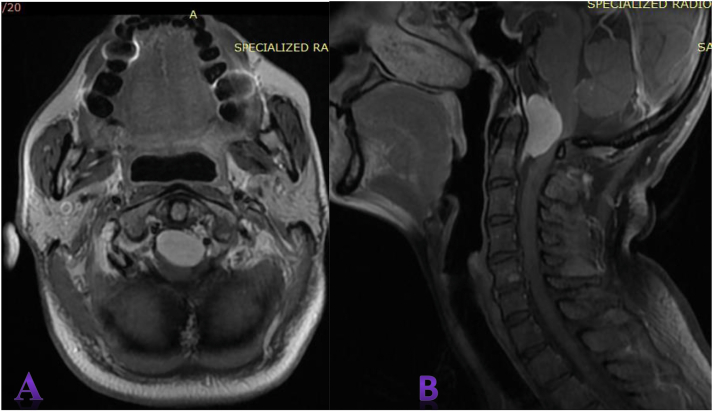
A: Post-contrast T1 weighted axial image, B: Post-contrast T1 weighted sagittal image: magneticresonance imaging (MRI) showing ventral placed, homogenously enhancing dural based lesion in the foramen magnum encasing both vertebral arteries suggestive of meningioma.
